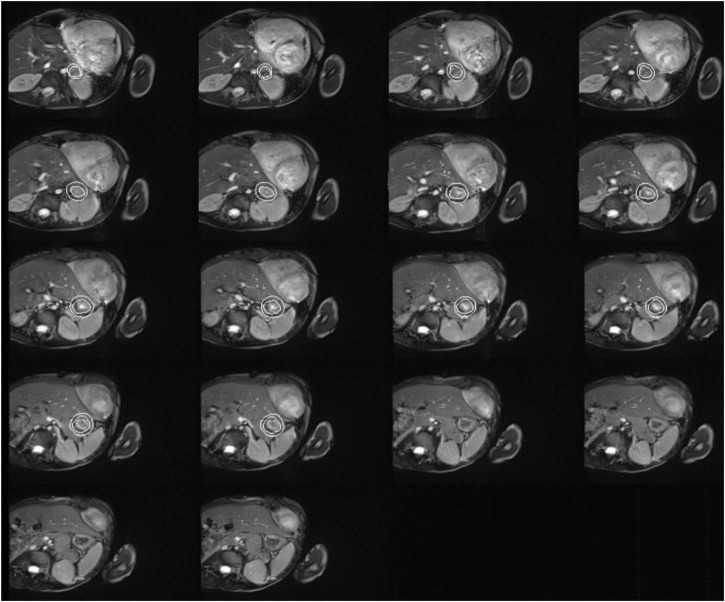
Cardiac magnetic resonance imaging of the patient with both dyschromatosis symmetrica hereditaria (DSH) and Aicardi-Goutières syndrome type 6 (AGS 6). Cardiac MRI image indicated mitral valve stenosis (MS), severe mitral regurgitation (MR), tricuspid regurgitation (TR), biatrial enlargement with the more significant left atrium, biventricular hypertrophy, and a small amount of pericardial effusion (PE).
